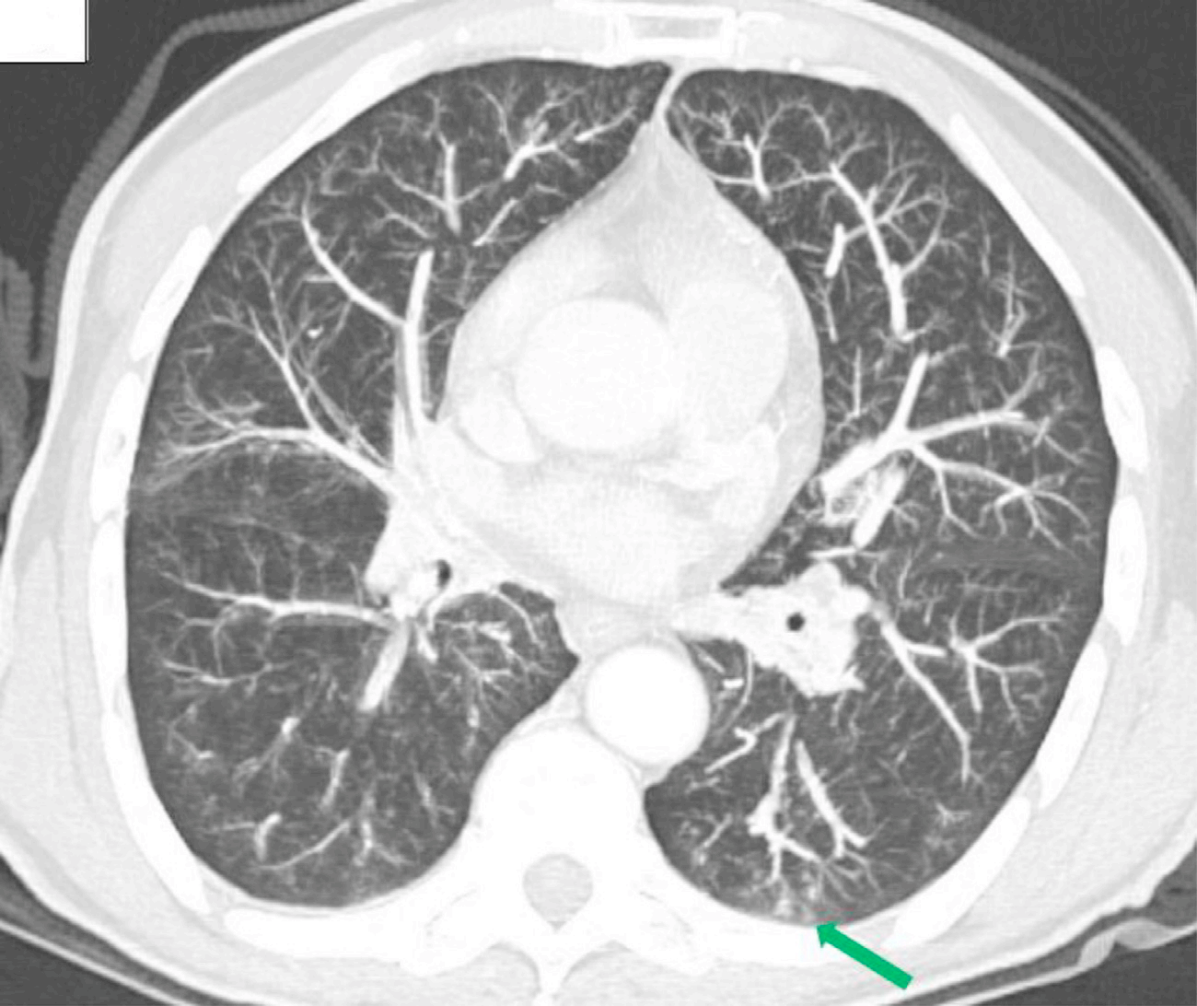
Thoracic CT scan. Axial slice in lung window. A cluster of bronchiolar micronodules forming a tree-in-bud pattern in the left Fowler, suggestive of an infectious bronchiolitis focus (green arrow).
